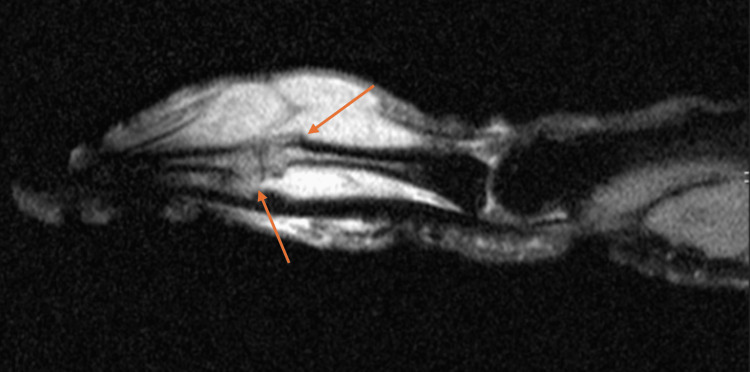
Lateral MRI image of the second finger. Arrows pointing to synovitis and bone injury on the proximal and middle phalanxes.
